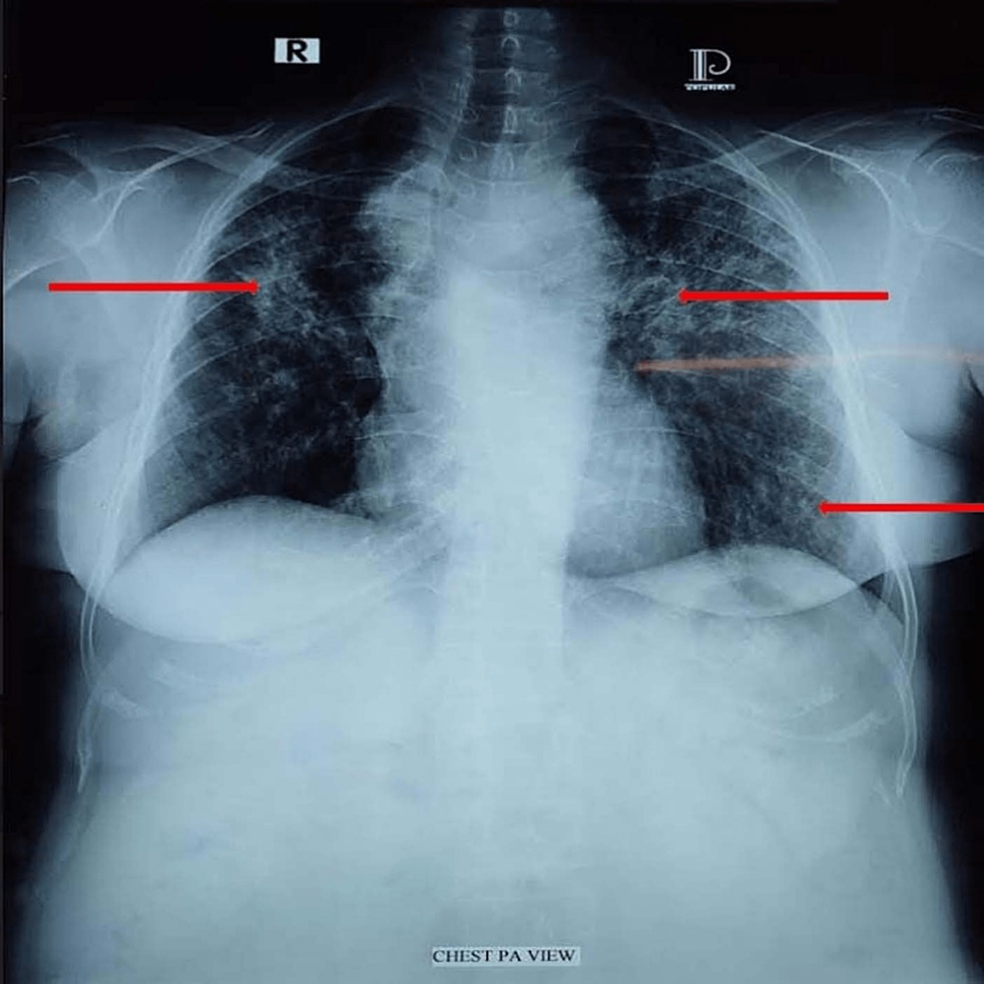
Anteroposterior chest X-ray image showing homogeneous opacities over the upper, middle, and lower zones of both lung fields
